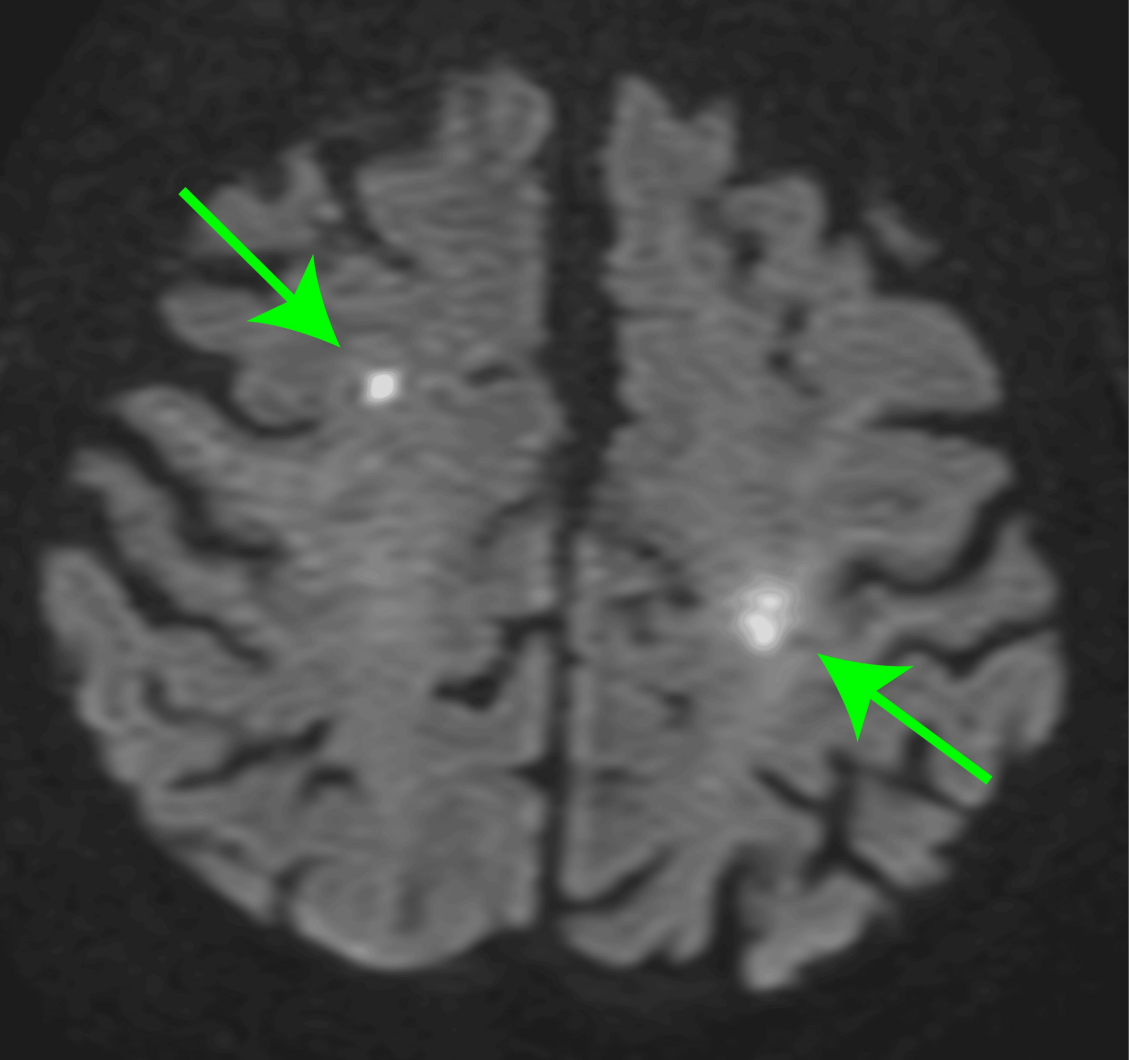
Magnetic resonance imaging of the head Diffuse cerebral infarct lesions in the bilateral cerebrum (green arrows).
